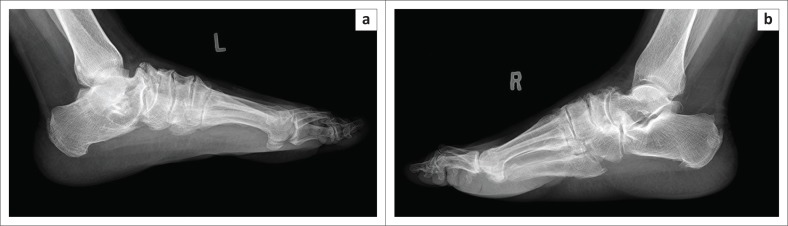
Lateral radiographs (a and b) of both feet in an HIV-positive patient. The radiograph demonstrates periostitis and enthesitis affecting the right foot more than the left foot.
